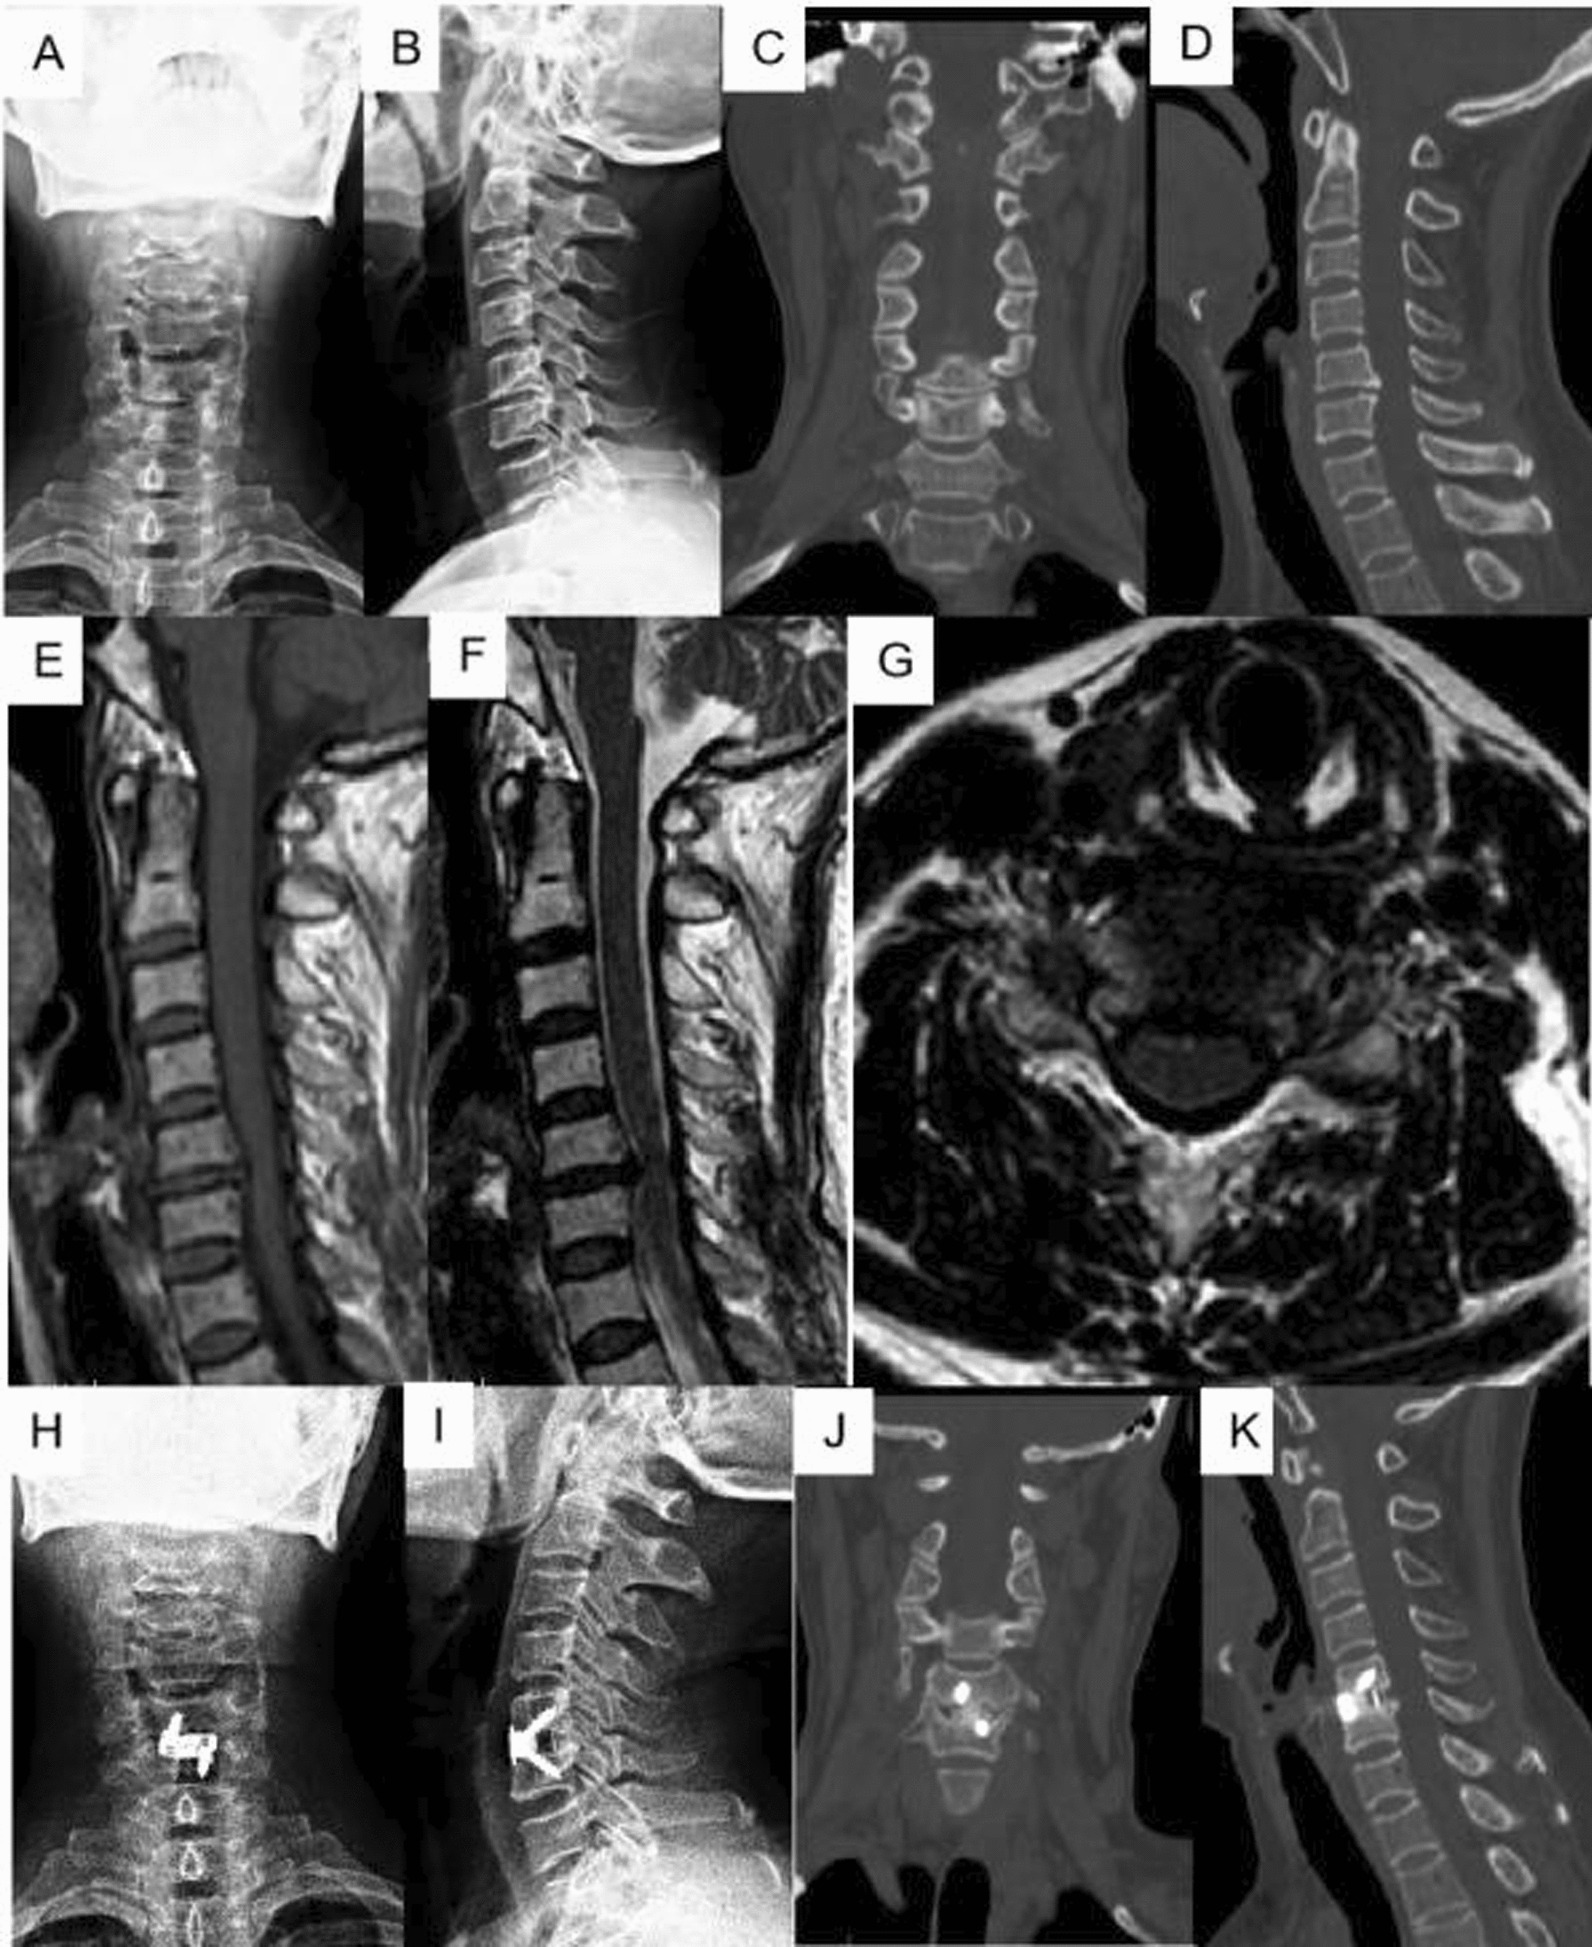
The patients underwent ACDF with ZERO-P VA internal fixation system. A Anteroposterior and B lateral of standing cervical X radiographs. C, D CT in 3D-sagittal reconstruction; E, F T1- weighted and T2-weighted MRI in sagittal plain, G MRI in axial plain of C5/6. Postoperative 6 months, H anteroposterior and I lateral of standing cervical X radiographs; J, K CT in 3D-sagittal reconstruction
